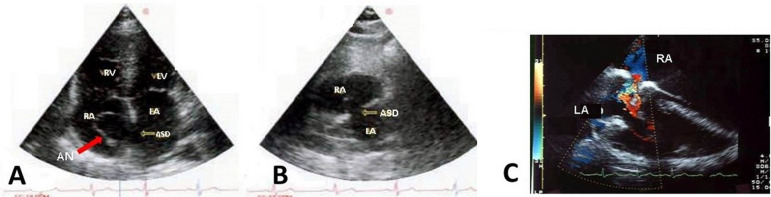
Transthoracic echocardiogram. A) The red arrow points to the atrial septal aneurysm; B) the arrow points to the atrial septal defect (ASD); and C) the echo Doppler shows the ASD flow. AN=aneurysm; LA=left atrium; LV=left ventricle; RA=right atrium; RV=right ventricle.
